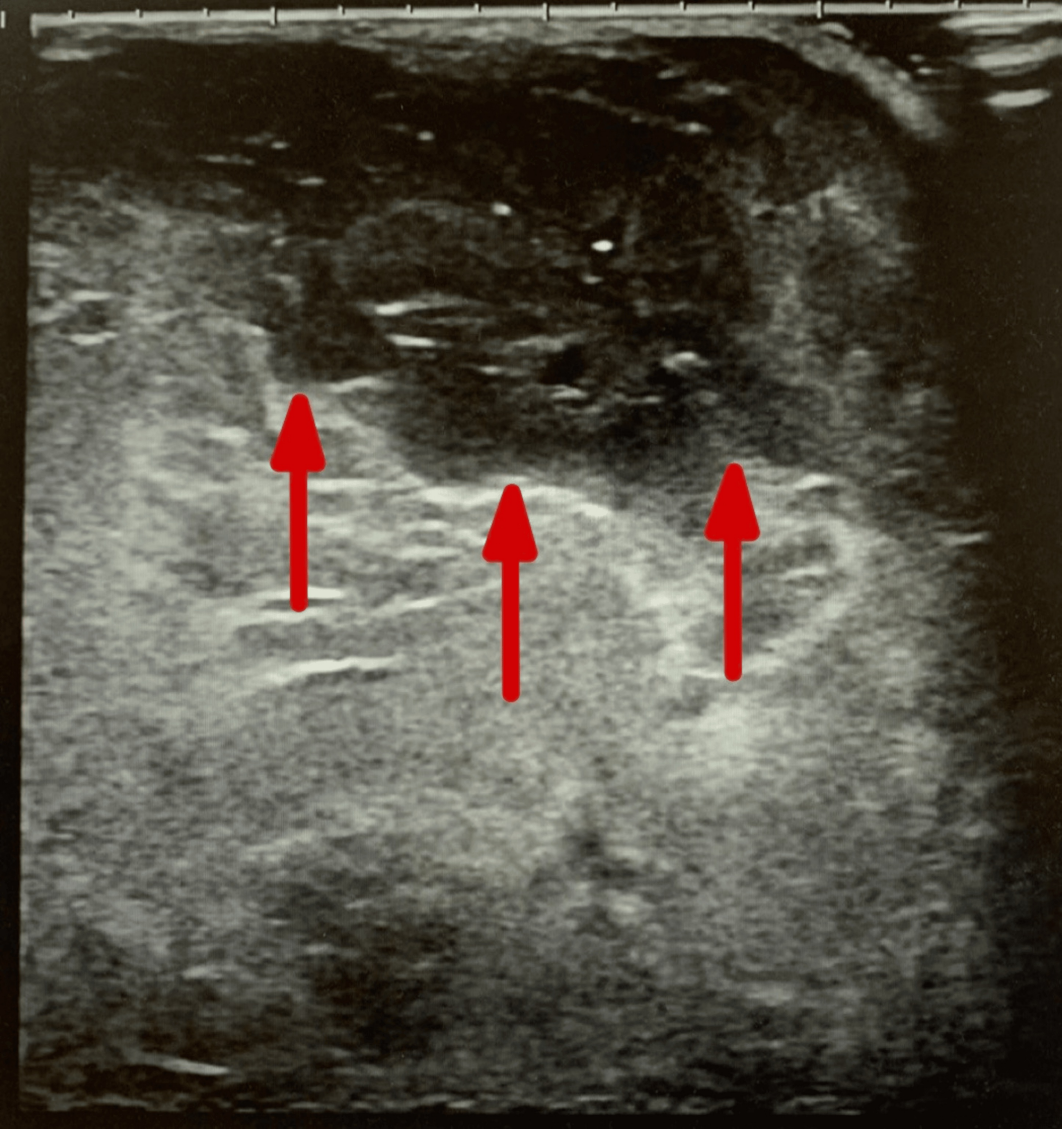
Preoperative ultrasonography of the patient
